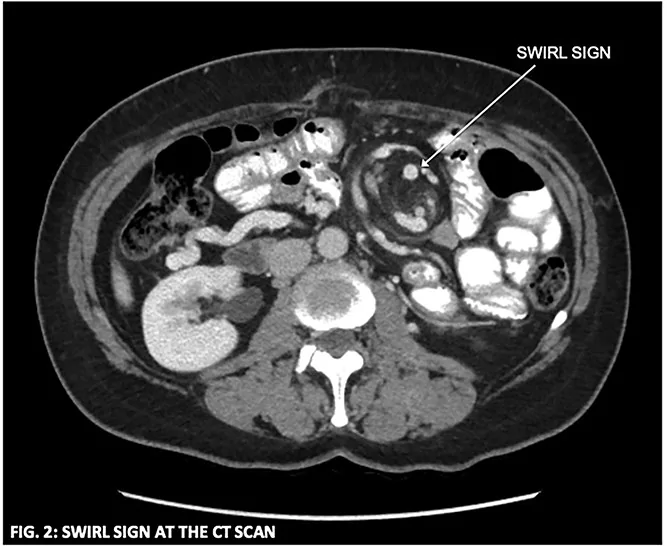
The “swirl sign” at CT scan.
